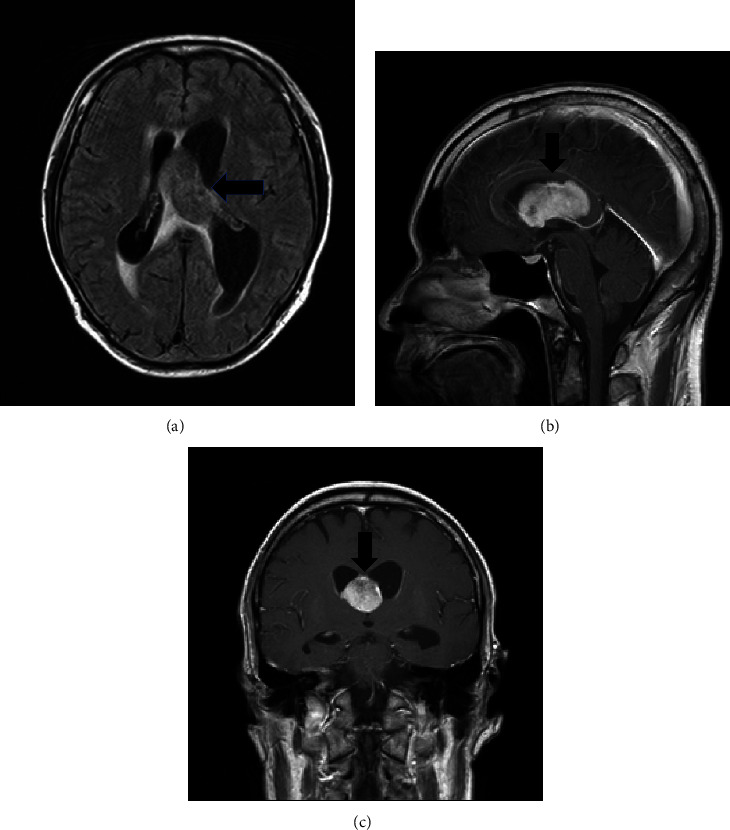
Magnetic resonance imaging (a)–(c) revealed a 4 cm lesion in the corpus callosum (indicated by the arrows). Panel (a) shows a fluid-attenuated inversion recovery image; (b) and (c) are T1-weighted images.
